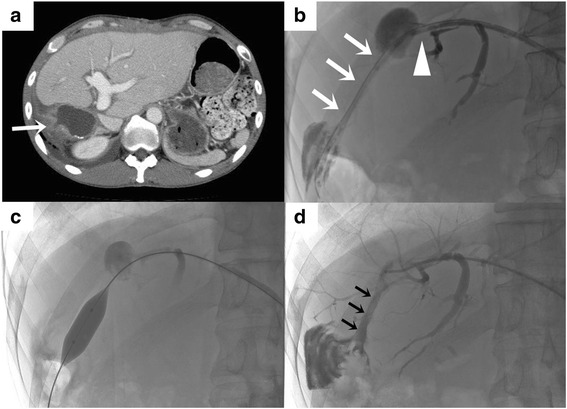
Stenosis of Roux limb at a month after discharge and the treatments. a CT showed dilatation of both the limb and intrahepatic bile ducts. *Arrow* indicates stenosis of the limb. b Tube cholangiogram through a PTBD tube of 12 Fr inserted across the stenotic lesion of Roux limb. Arrows indicate stenosis of the limb. The triangle arrow indicates the intact hepaticojejunostomy anastomosis. c Balloon dilation of the stenotic portion of the limb (12 mm in diameter). d The PTBD tube was removed 2 years after the emergency event. Arrows indicate the expanded limb
